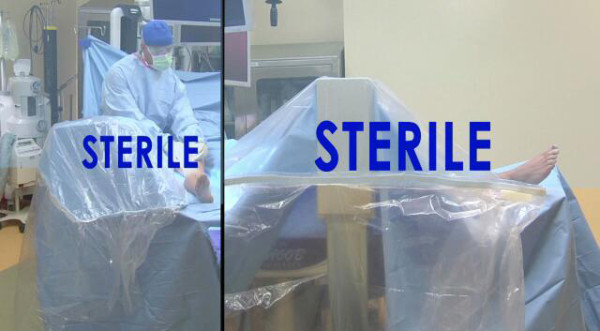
Five sided protection. C-armor adheres to equipment draping standards covering the x-ray tube on the top, and all four sides with a sterile barrier.
